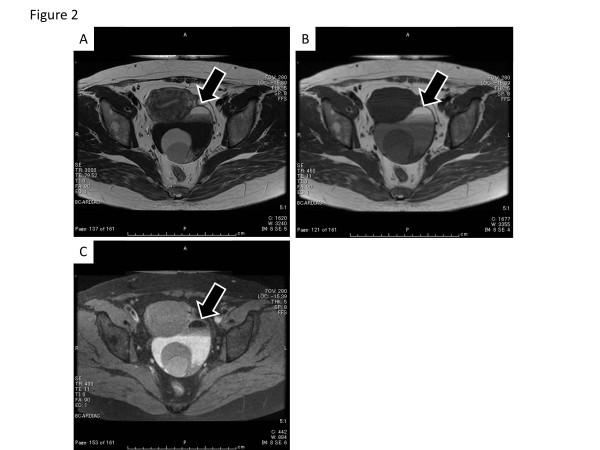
MRI findings before surgery. Horizontal T2-weighted (A) and T1-weighted (B) MR imaging demonstrated a fat component, which showed a drop in the signal intensity on fat saturated T1-weighted images (C) in the multilocular cystic lesion without a solid part (arrow).
